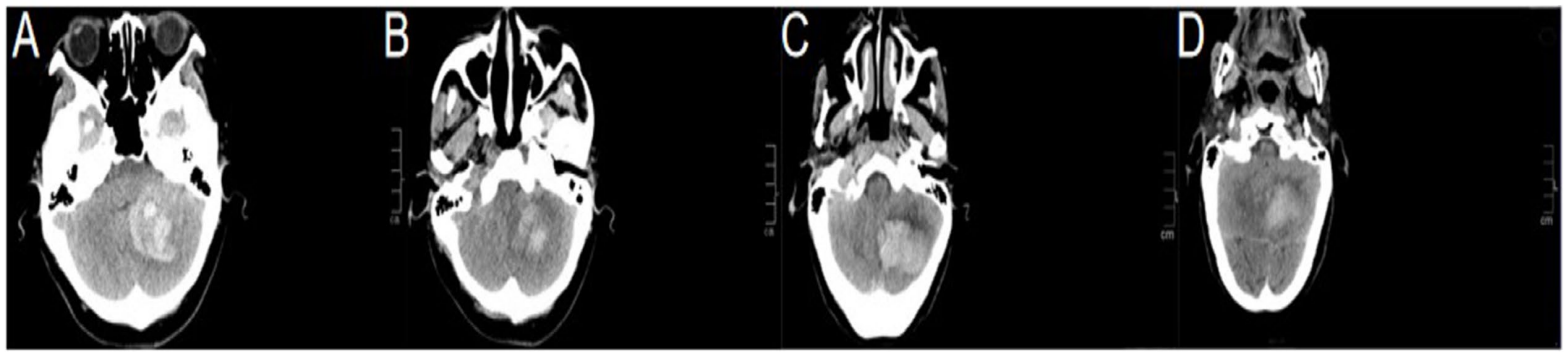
(A–D) All brain computed tomography scan results before and after conservative treatment.
